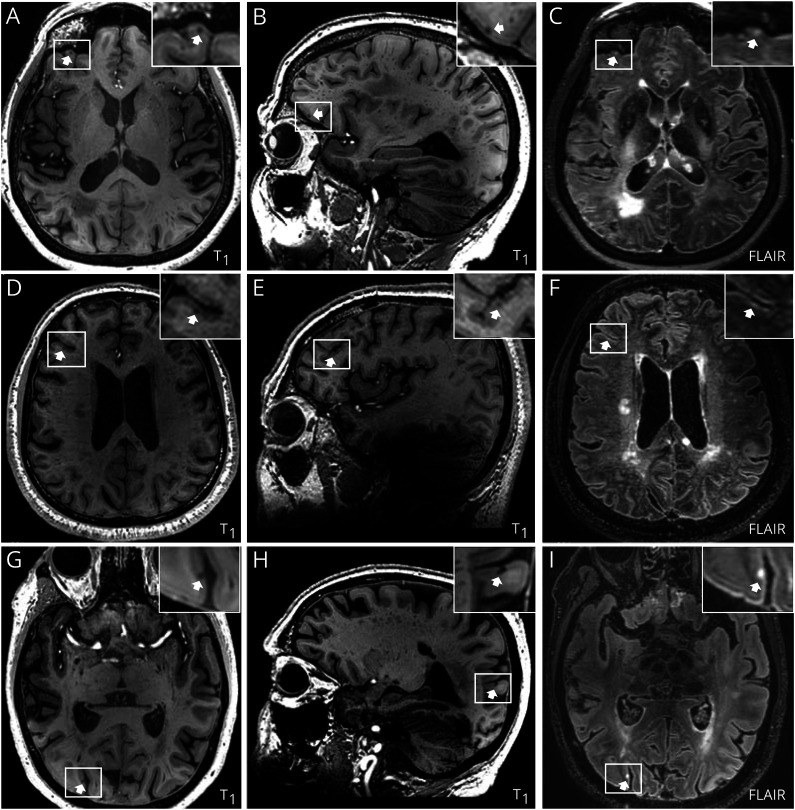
Examples of CMIs in Patients With sICH Panels A–C show the 7T MRIs of a patient with a right lobar sICH, fulfilling the Boston criteria version 2.0 of probable cerebral amyloid angiopathy, and a CMI (white arrows) in the superficial part of the cortex (A: axial T_1_-weighted image; B: sagittal T_1_-weighted image; C: axial FLAIR image). Panels D–F depict a patient with a left deep sICH and a CMI (white arrows) in the deeper part of the cortex (D: axial T_1_-weighted image; E: sagittal T_1_-weighted image; F: axial FLAIR image). Panels G–I show images of a patient with a right deep sICH and a transcortical CMI (white arrows; G: axial T_1_-weighted image; H: sagittal T_1_-weighted image; I: axial FLAIR image). The inserts show the CMIs in more detail. CMI = cerebral microinfarct; FLAIR = fluid-attenuated inversion recovery; sICH = spontaneous intracerebral hemorrhage.
